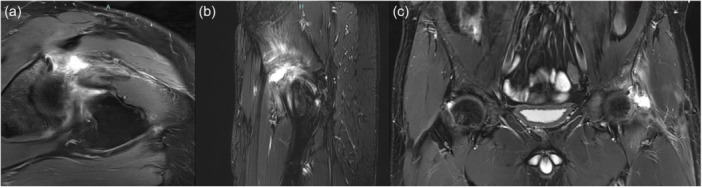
Postoperative magnetic resonance imaging (MRI) of the pelvis demonstrating a capsular defect of the left hip following a postoperative fall within 90 days of left hip arthroscopy for FAIS. (a) Axial, (b) sagittal and (c) coronal sequences show focal discontinuity and signal hyperintensity consistent with a capsular rupture on the left side. Both hips had previously undergone hip arthroscopy, allowing for direct comparison with the contralateral (right) side, which demonstrates intact capsular tissue on the coronal image. FAIS, femoroacetabular impingement syndrome.
